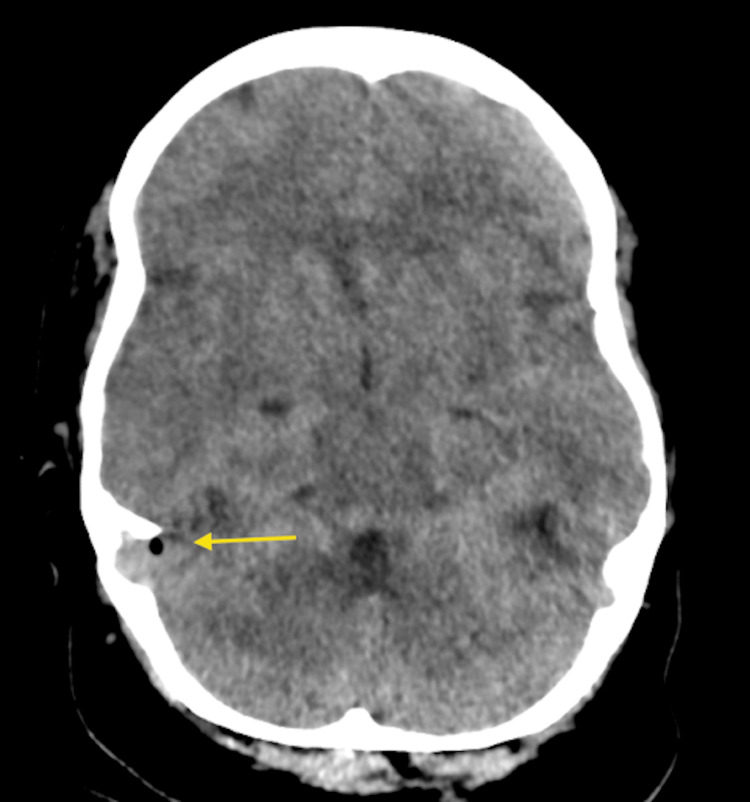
Non-contrast CT Head (axial view) demonstrating locules of intracranial gas consistent with pneumocephalus (arrow), located adjacent to the right sigmoid sinus.
